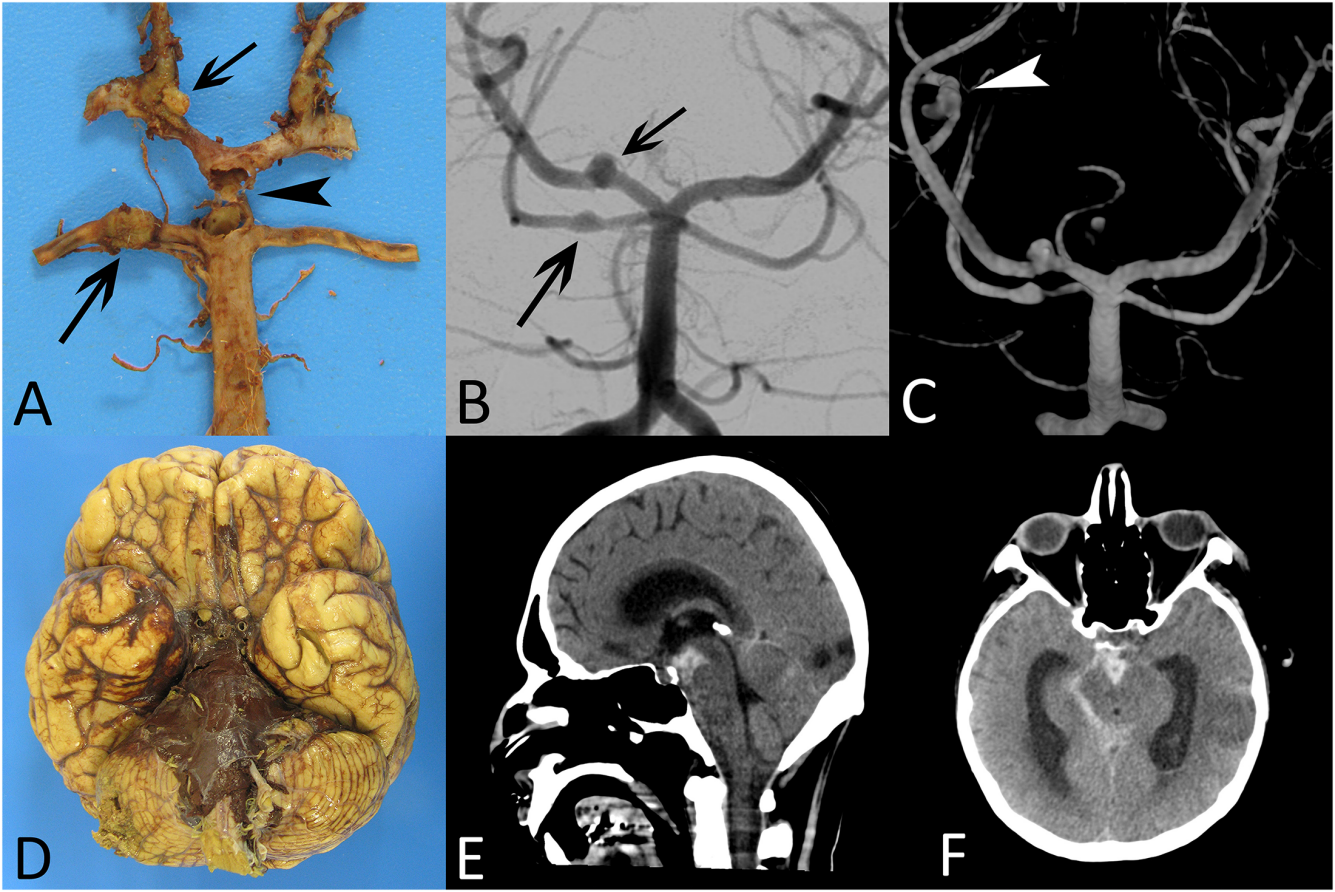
Macroscopic and imaging characteristics of vascular complications (patient #13). Gross examination (A) and cerebral angiogram (B) show aneurysmal lesions on superior cerebellar artery, posterior cerebral artery (arrows) and a ruptured aneurysm of the distal part of the basilar artery (arrowhead). The 3D angiography (C) shows an additional distal fusiform aneurysm on the middle cerebral artery (arrowhead). Massive cerebral hemorrhage into the basal cisterns (interpedoncular and pontine cisterns) visualized on gross examination (D) and non-enhanced CT scan (E and F).
